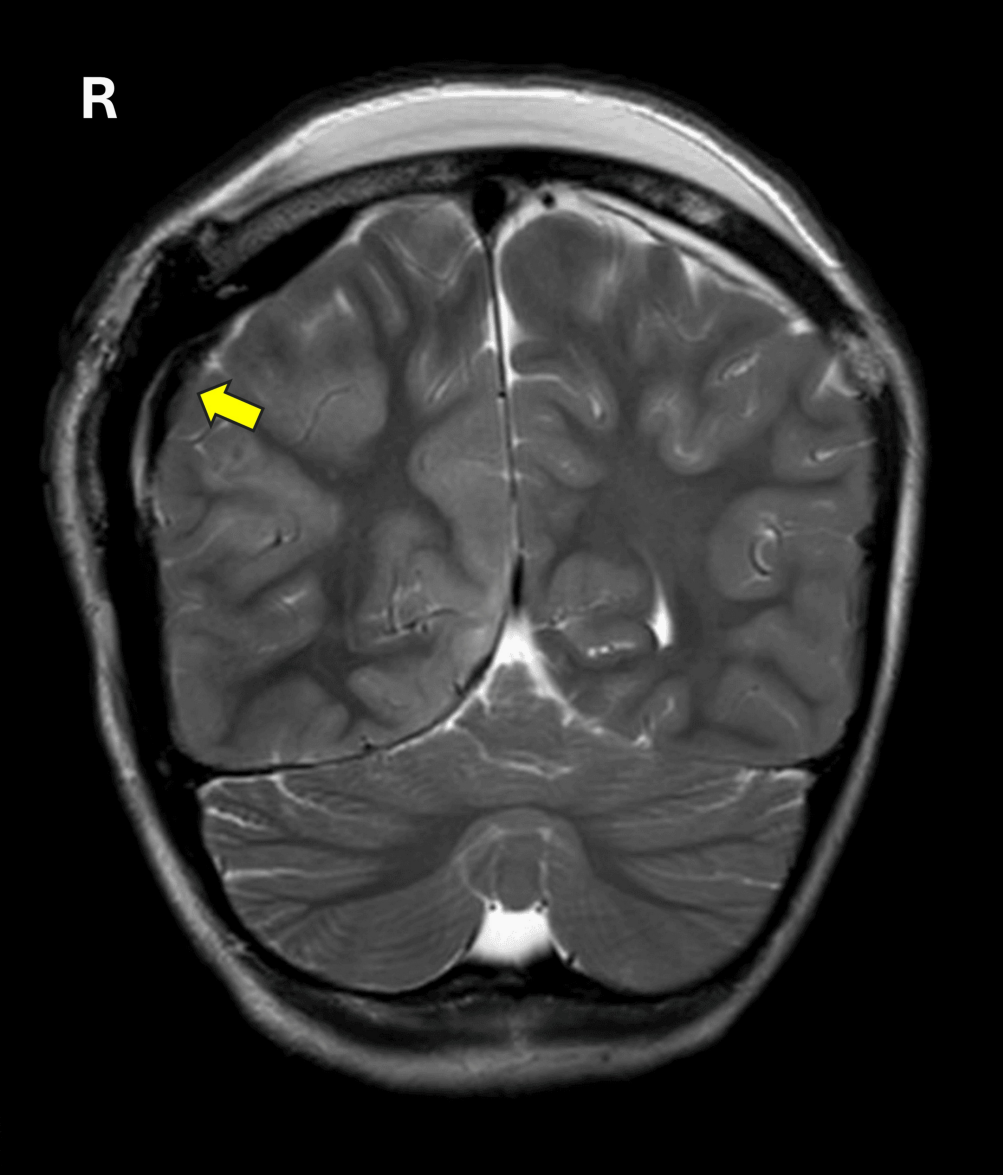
MRI examination of case 2 after evacuation of the extradural hematoma. Coronal T2 sequences demonstrate altered bone marrow signal intensity, involving the right and left sides of the skull. There is an associated subgaleal collection seen posteriorly, which is highly suggestive of acute soft head syndrome.
